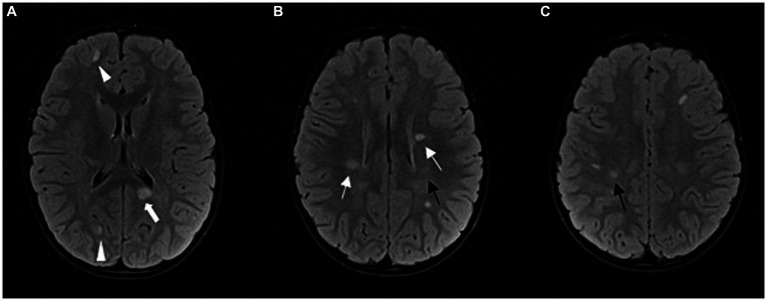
Follow-up MRI after 1 year: FLAIR sequence in axial plane at three different levels (A–C); progression of the disease; multiple demyelinating lesions in typical periventricular (arrows) and juxtacortical locations (arrowheads). Among the periventricular lesions, we can observe a lesion in the splenium of the corpus callosum on the left side (thick arrow). Other new lesions can also be observed in the subcortical and deep white matter of the frontoparietal lobes. The lesions shown in Figure 1 have partially regressed (black arrows).
